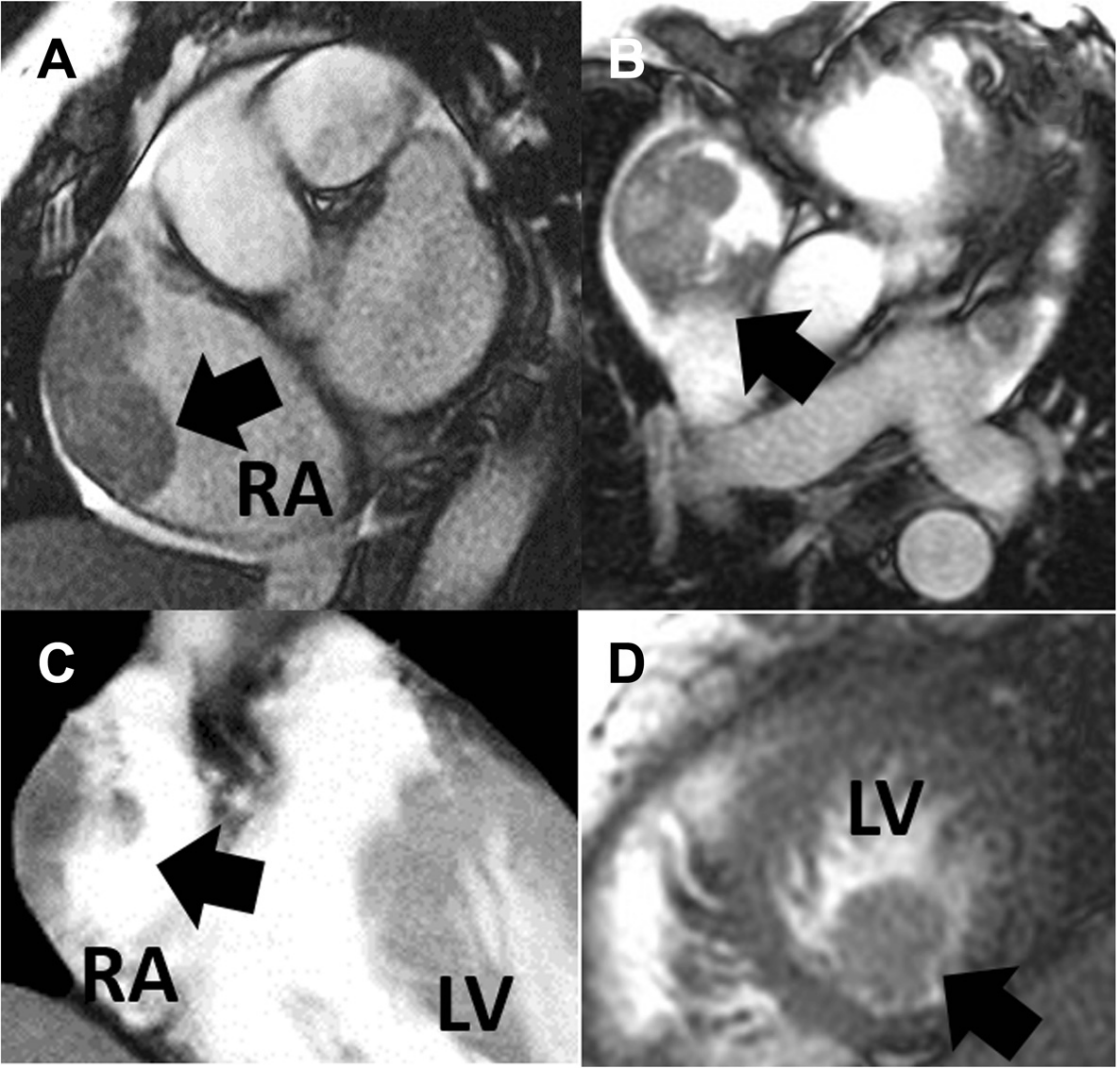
On cardiac magnetic resonance imaging (MRI) as cardiac-gated, cine gradient-echo “bright blood” magnetic resonance images, one 6.0 × 2.3cm lobulated mass bulging out from the lateral wall of the right atrium (RA) (a, b, c; *black arrows*) and another small nodule in the left ventricular apex (d; *black arrow*). LV: left ventricle
